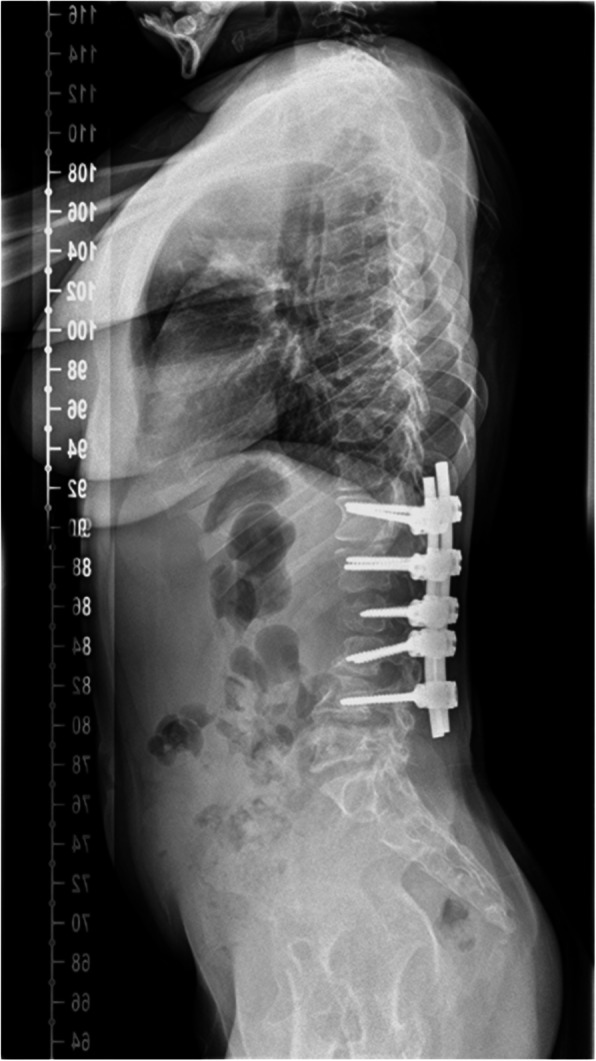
Post-operative radiograph of the same patient after correction of thoracolumbar kyphosis- Lateral view
